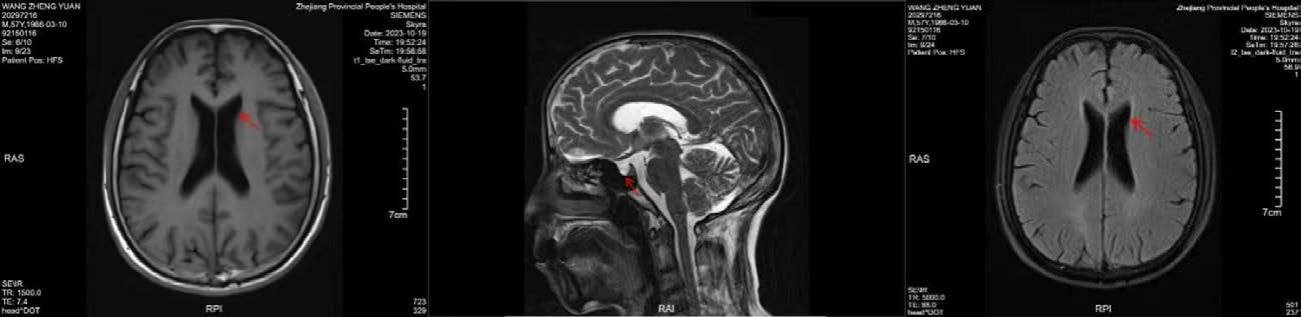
Contrast-enhanced head MRI showing a high signal in the white matter. With nonspecific abnormal signals on MRI, the possible locations of the lesions could be identified. Therefore, further analysis must be performed to validate the diagnosis. MRI = magnetic resonance imaging.
